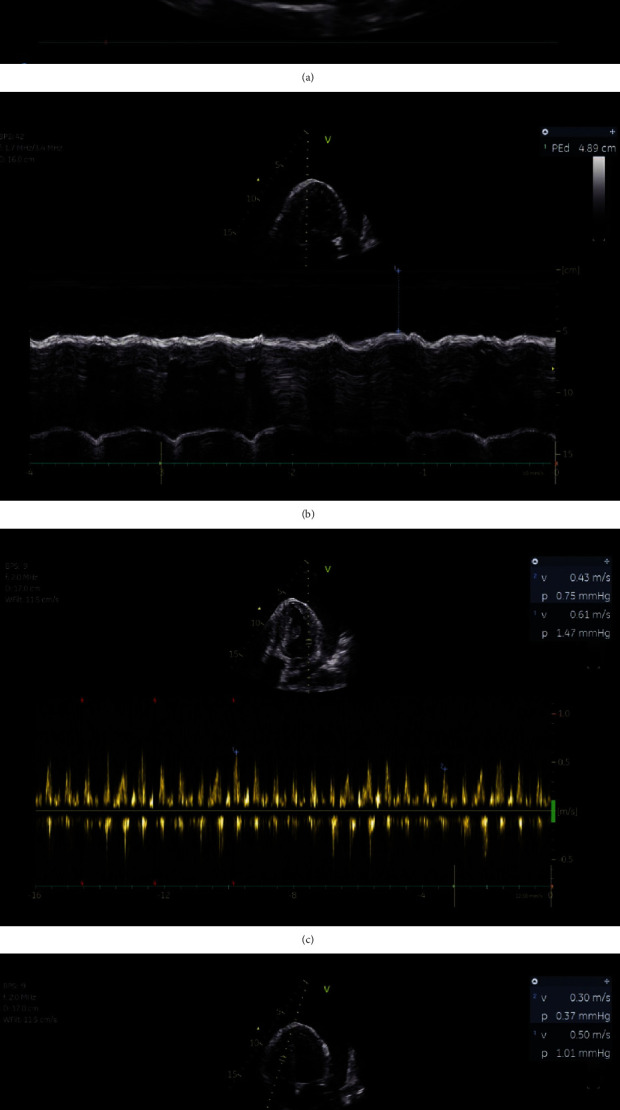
Initial transthoracic echocardiography indicating pericardial tamponade. (a) Apical 4-chamber view with large circumferential pericardial effusion. (b) Apical 4-chamber view (M-mode) with 4.89 mm pericardial effusion end diastolic. (c) Mitral inflow respiratory variation > 25% and (d) tricuspid inflow respiratory variation > 40% by PW-Doppler indicating tamponade morphology; scale and velocity settings are not optimal due to intensive care unit bedside echocardiography.
